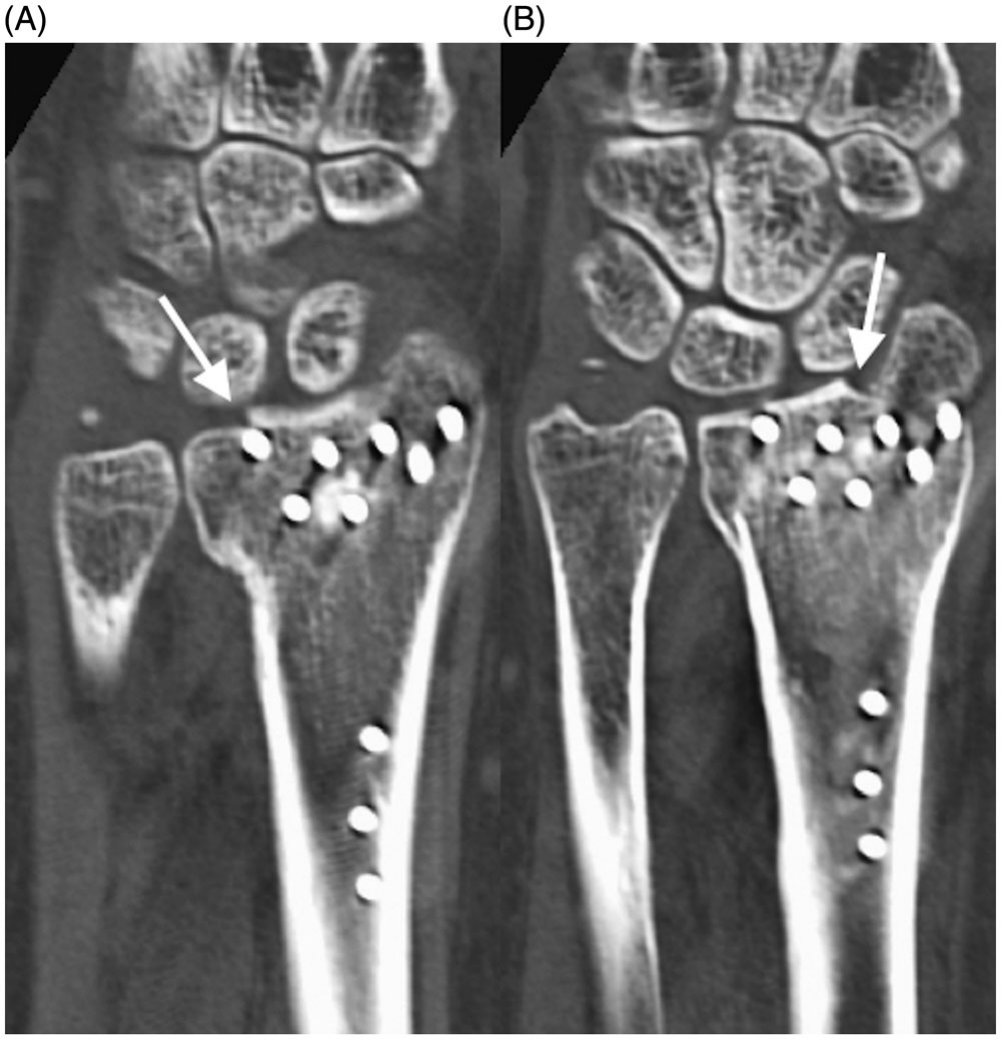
CT images showing an irregular articular surface due to poor reduction in the initial surgery.
